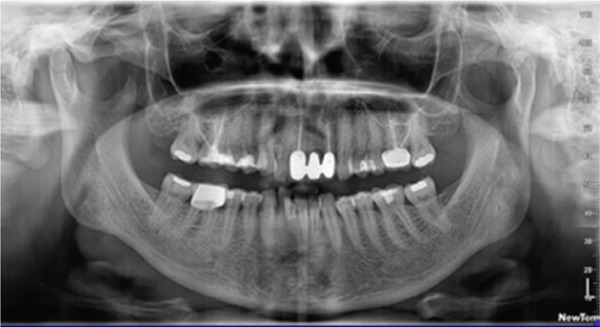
Orthopantomogram (OPT) radiograph showing the panoramic view of the patient′s dental structures.
